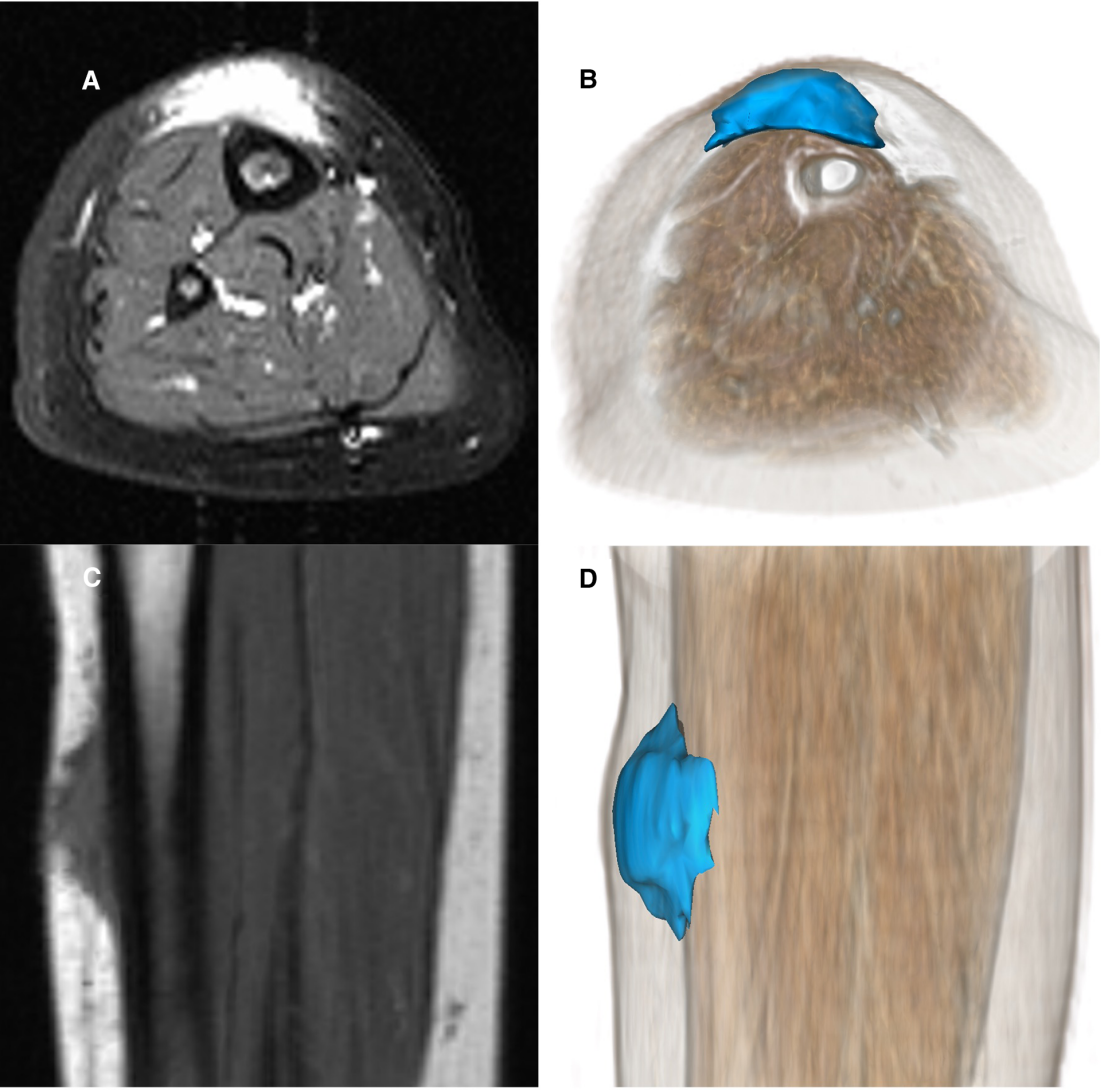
Representative MRI sections and 3D-reconstructions of subcutaneous granuloma annulare lesion (colored blue) on the right lower leg of a 2.5-year-old girl (A–D). A T2-weighted slice in axial orientation (A) shows the cap-shaped epifascial lesion with high signal intensity (bright). The corresponding 3D reconstruction is given in (B), visualizing the lesion in blue color and the remaining other tissues by overlaying a volume rendering in a skin-like color. (C) shows the lesion in a T1-weighted sagittal slice with low signal intensity (dark). The corresponding 3D rendering (D) demonstrates the cap-shaped morphology, extending from the deep fascia to the subcutaneous layer.
